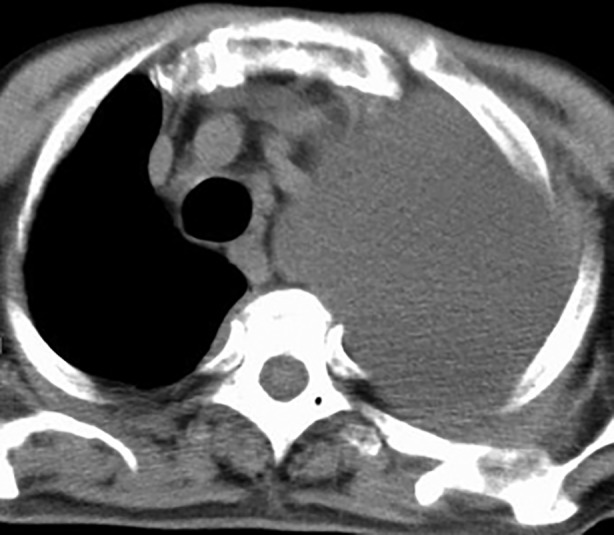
The patient's computed tomography scan of the chest showed large left‐sided pleural effusions.
